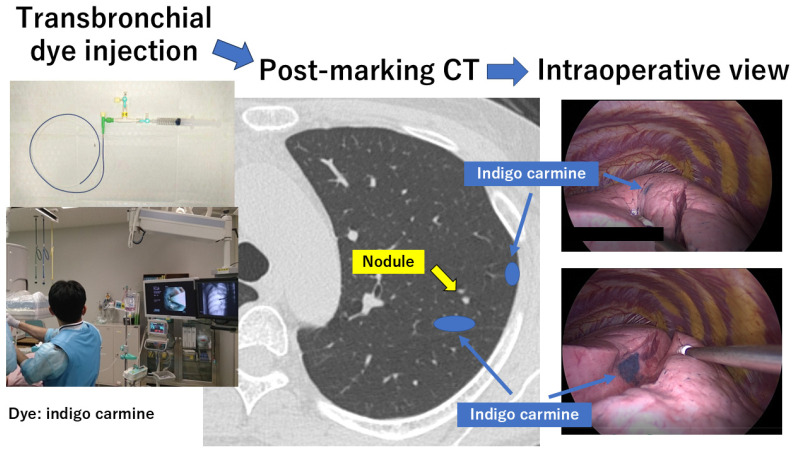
Virtual-assisted lung mapping (VAL-MAP). CT: computed tomography.
